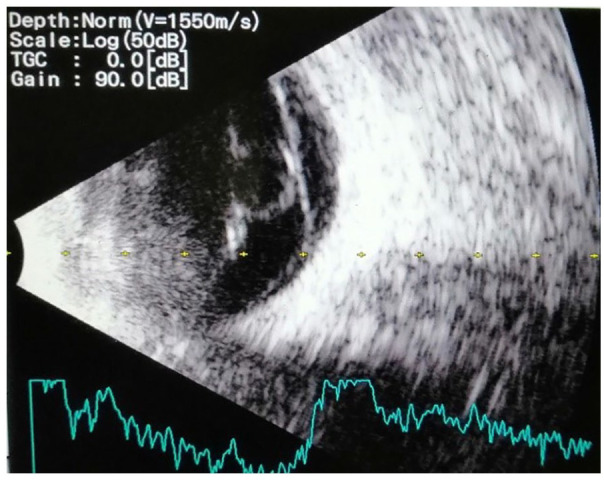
Left eye with tractional bands and a round intravitreal lesion. Scan of B-ultrasonography of patients orbits.
